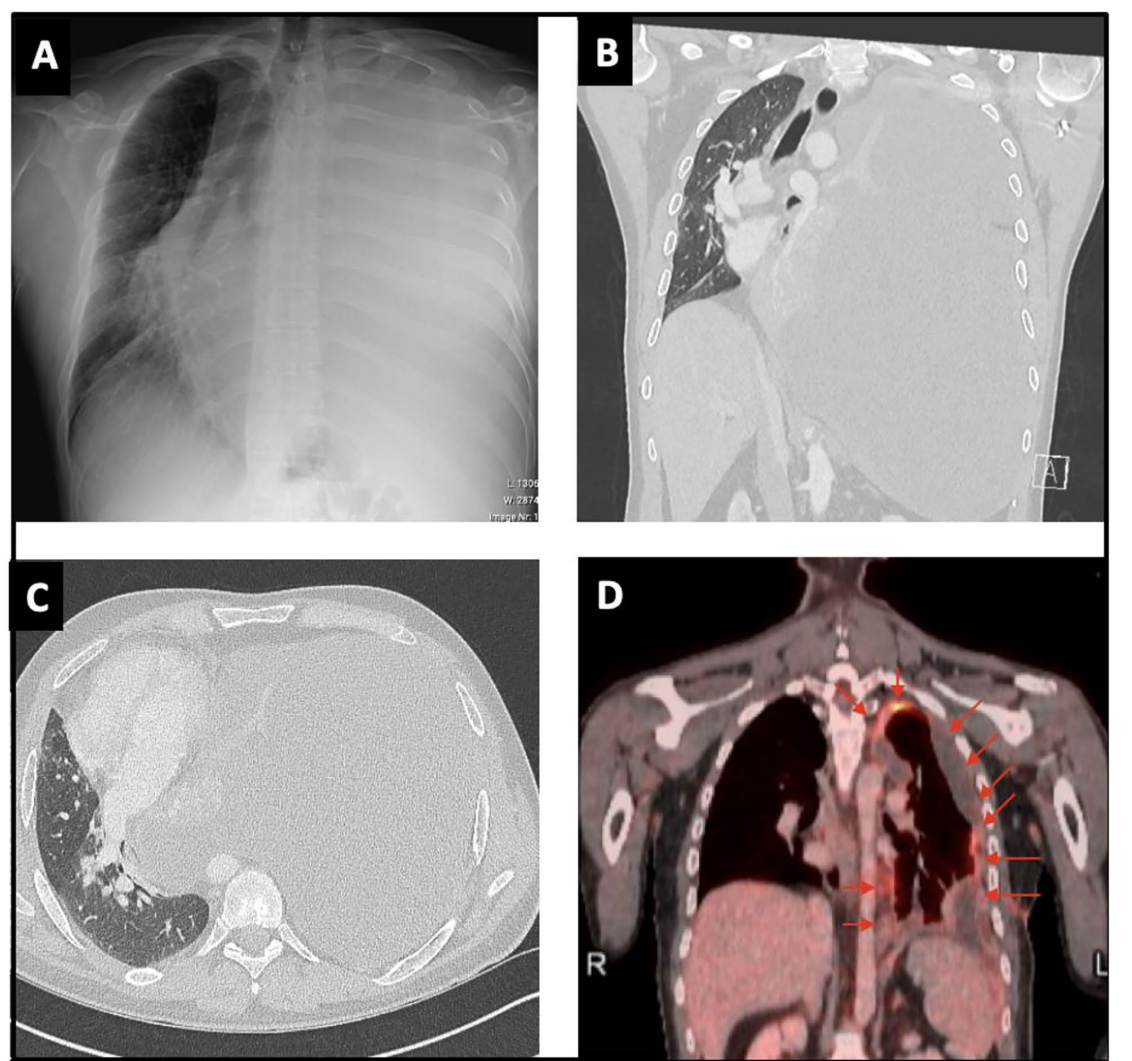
(A, B, C) Chest x-ray and CT scan showing a large left-sided pleural effusion with abnormal pleural thickening; (D) PET-CT prior to neoadjuvant chemotherapy showing diffuse pleural thickening (partially due to a recent talcage), but without extra-thoracic disease
